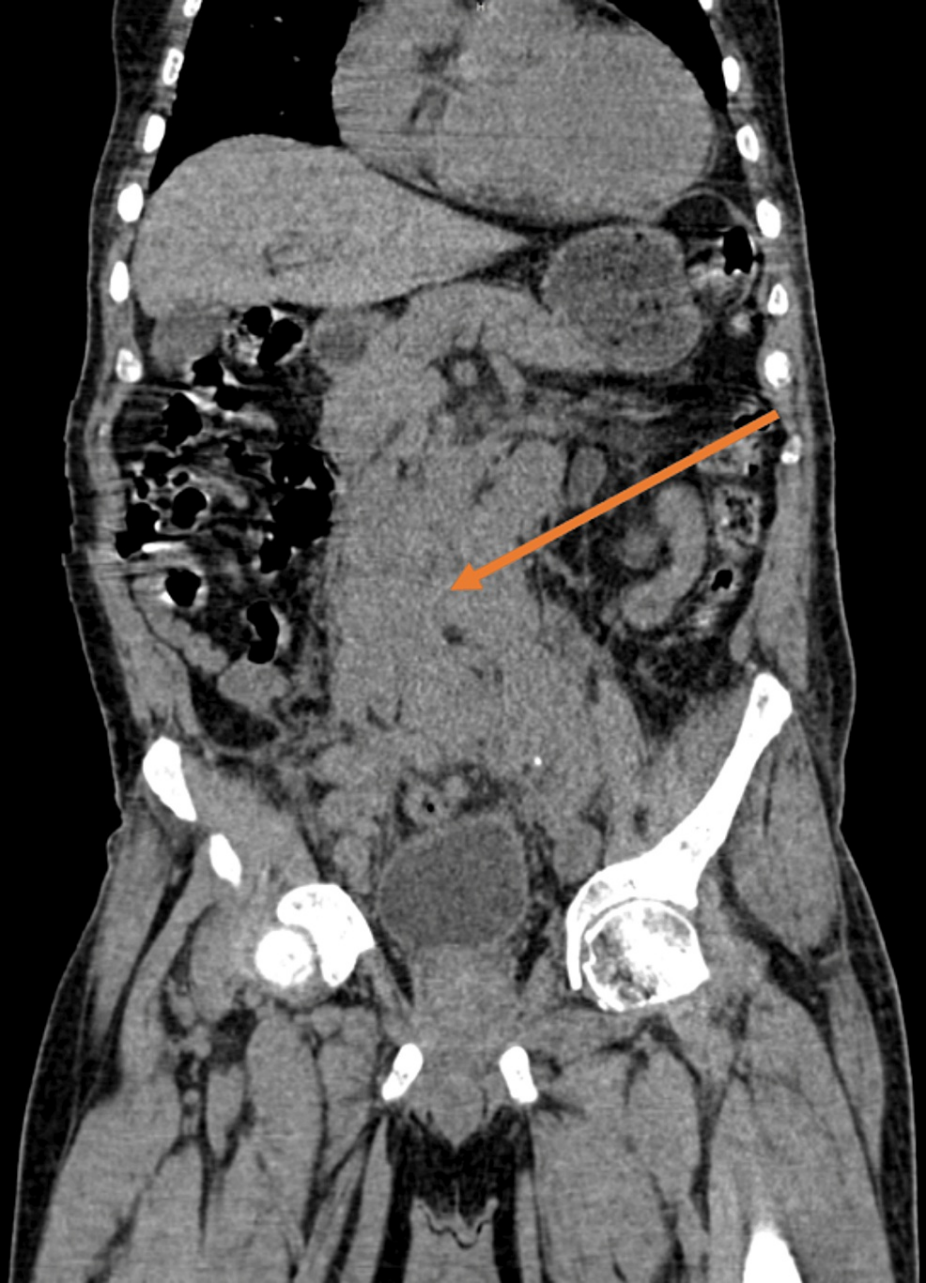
Coronal section of computed tomography of kidney, ureters and bladder indicating large hypodense mass (Orange arrow).
